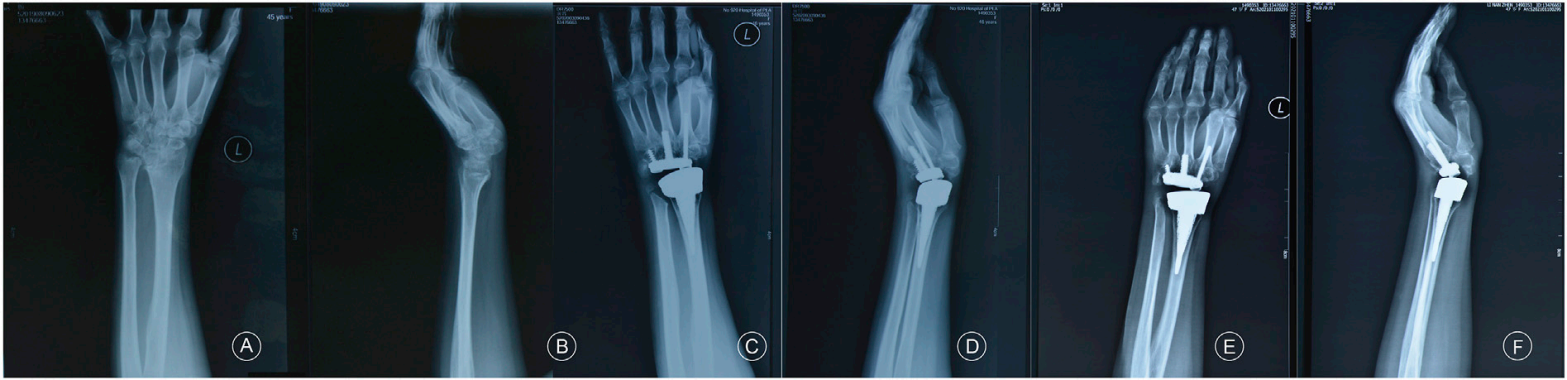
Osteoarthritis of the left wrist joint in a female patient aged 46 years. (A,B): preoperative anteroposterior and lateral radiographs, (C,D): postoperative anteroposterior and lateral radiographs at 6 months, (E,F): postoperative anteroposterior and lateral radiographs at 12 months.
